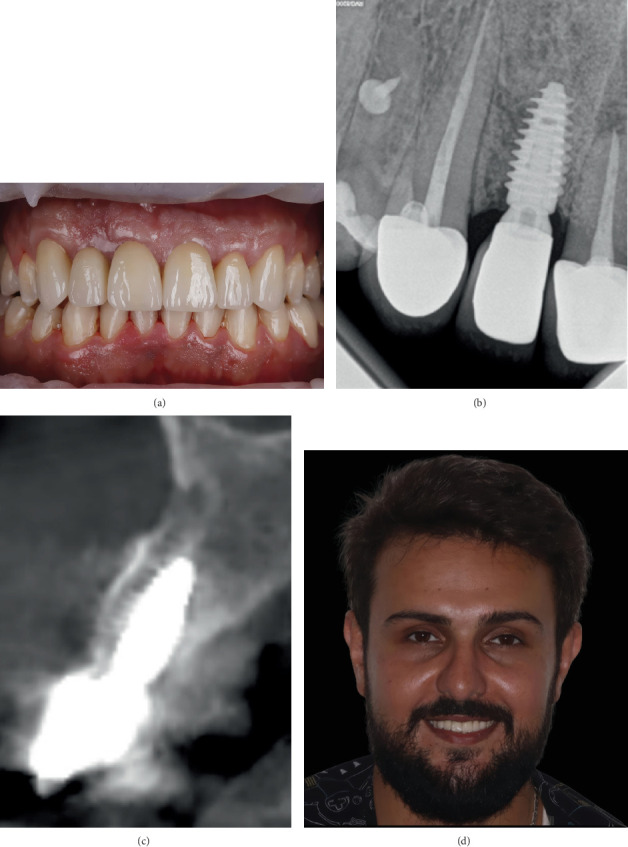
The postoperative situation. (a) Intraoral frontal photo showing the final esthetic outcome. (b) Peri-apical radiograph showing the area of the implant. (c) Sagittal cone beam computed tomography slices taken 12 months postoperatively. (d) Extraoral photo of the patient smiling.
